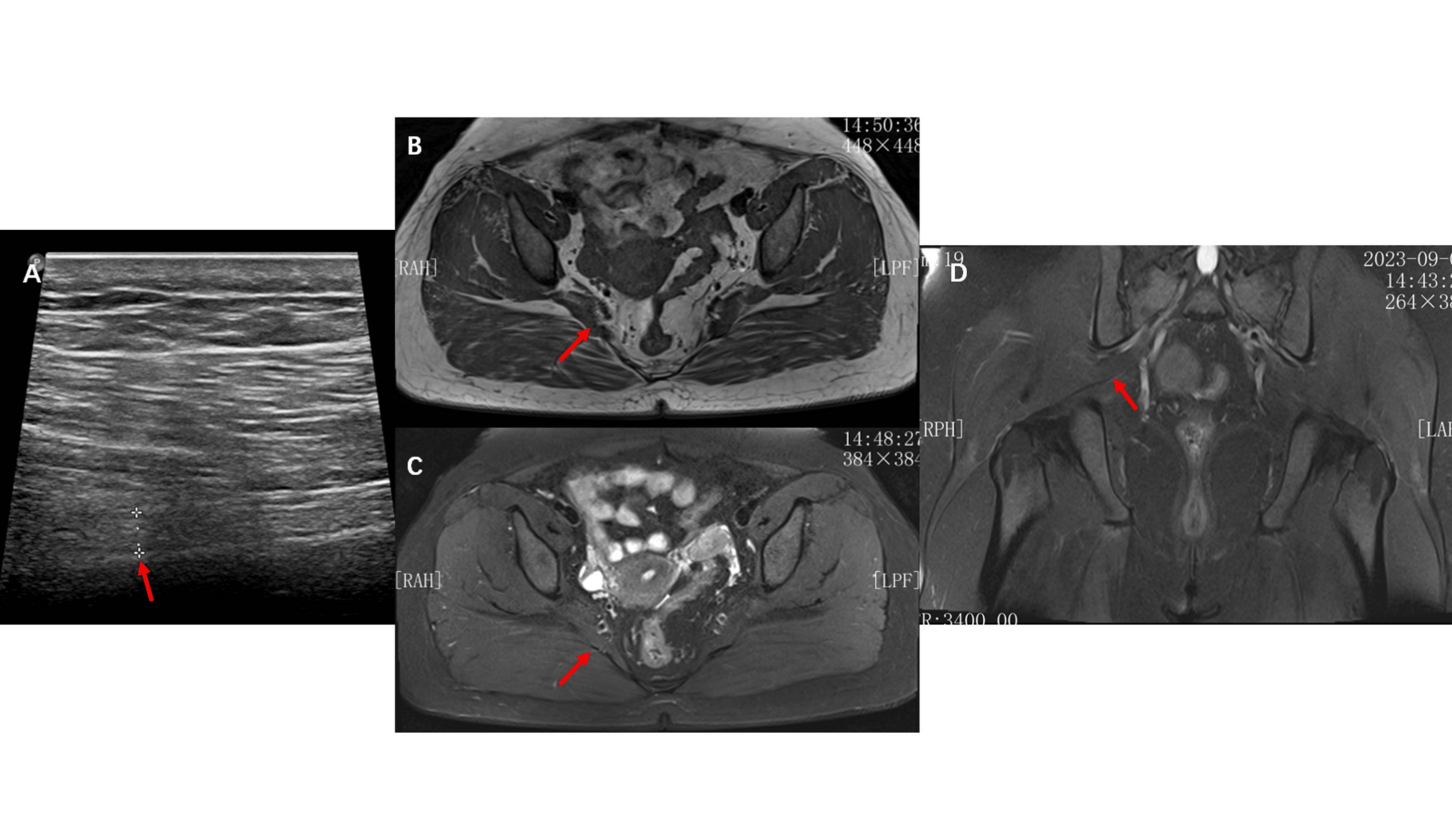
Doppler ultrasound examination of the sciatic nerve and MRI of the buttocks (A) Doppler ultrasound: sciatic nerve (red arrow). (B) MRI T1WI axial, (C) MRI T2W-FS axial, (D) MRI T2W-FS coronal. No abnormalities on MRI. The red arrow indicates the piriformis muscle.
